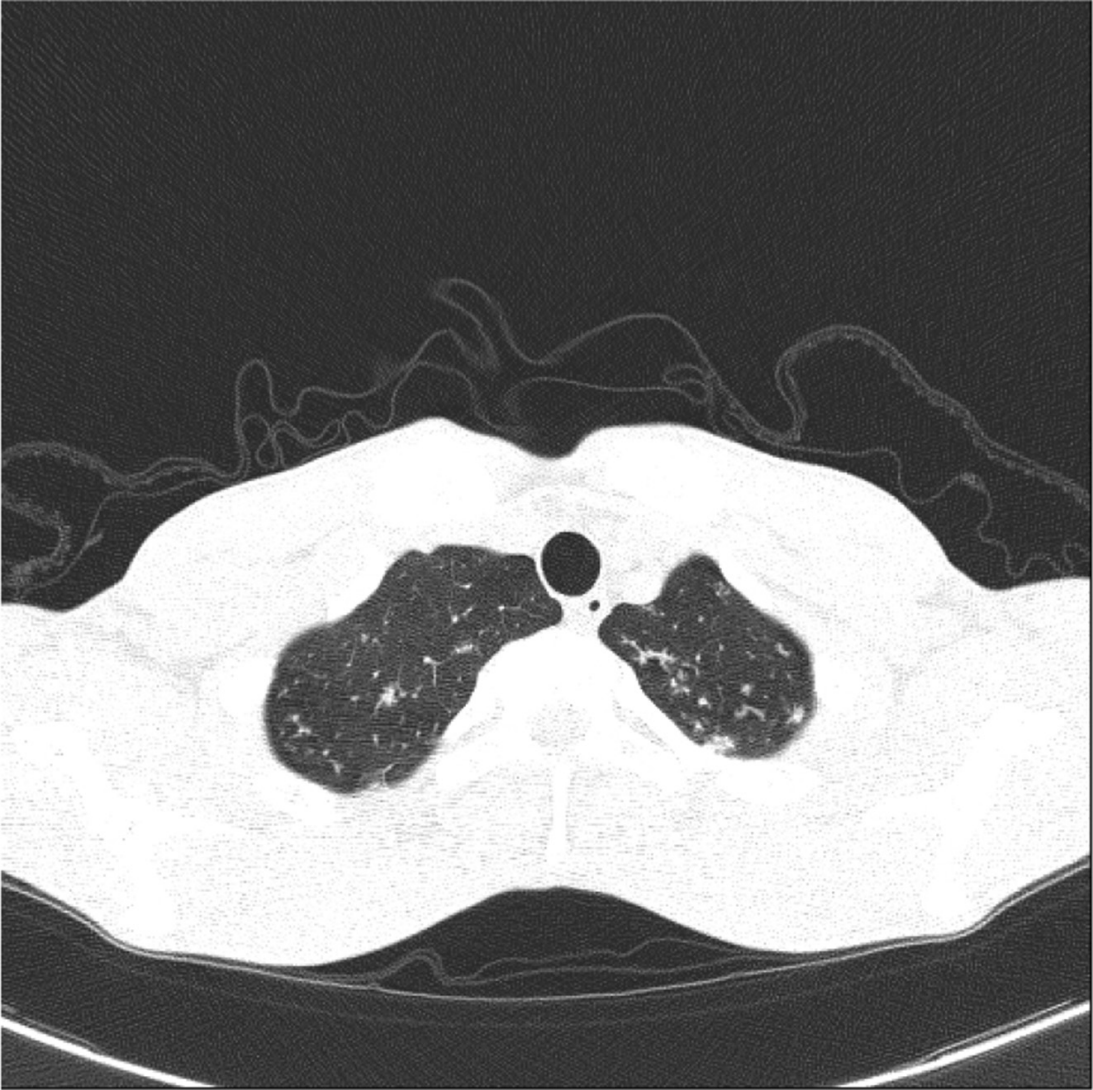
Chest CT scan. Tree in bud pattern in superior lobes.
